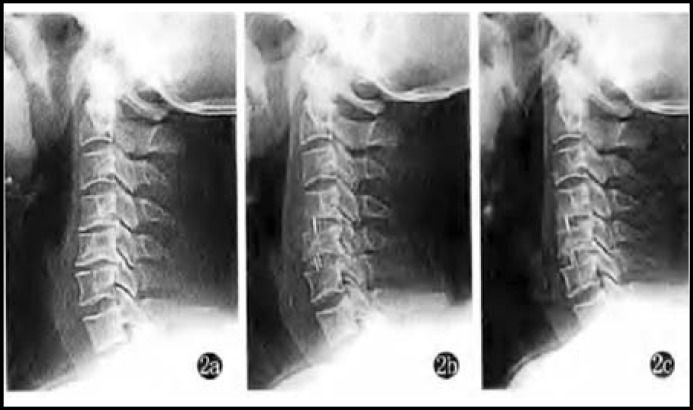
Mr. Liu, male, 62 years old, T_1_ segment. a: Preoperative X-ray film disclosed height of the injured vertebral body and Cobb angle; b: height of the injured vertebral body and Cobb angle immediately after anterior decompression; c: height of the injured vertebral body and Cobb angle three months after surgery (satisfactory maintenance and bone fusion).
